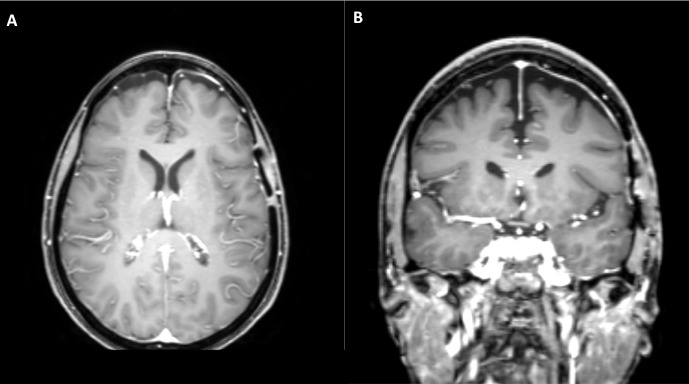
Postoperative magnetic resonance imaging of patient one A) Axial and B) coronal post contrast T1 images demonstrate gross total resection of the meningioma
